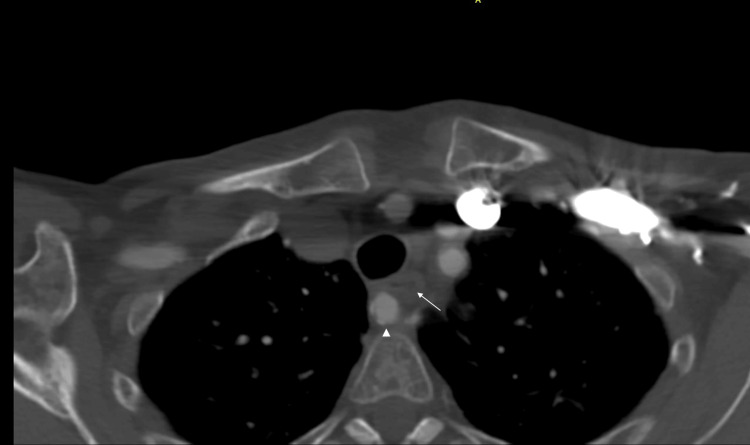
Axial view of CTA showing compression of oesophagus (white arrow) by the retroesophageal ARSA (white arrowhead) CTA: Computed tomography angiography, ARSA: Aberrant right subclavian artery
